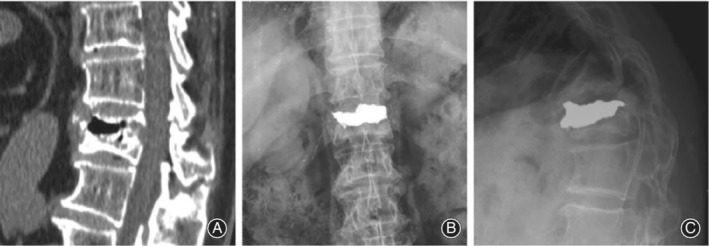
A typical patient with pattern I IVC from Wu *et al*.^16^. (A) Preoperative CT show the IVC near to the endplate.(B) cement leak into intervertebral disk. (C) adjacent vertebral body fracture 3 months after the first surgery.
